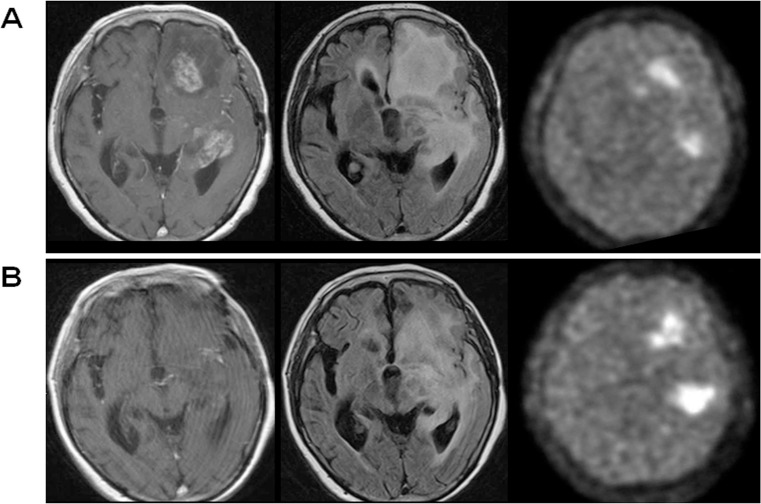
Representative cases of MRI-only responder (Case 2). Gadolinium-enhanced T1-weigted images (left panels), FLAIR images (middle panels), and FMISO PET images (right panels) are shown, and upper panels show the case before BEV treatment and lower panels show the case three courses after BEV administration. After BEV treatment, contrast-enhanced lesion and FLAIR hyperintensity lesion were significantly decreased, whereas FMISO accumulation was increased in both T/N ratio (from 3.70 to 4.81) and volume (from 33.5 mL to 38.5 mL).
